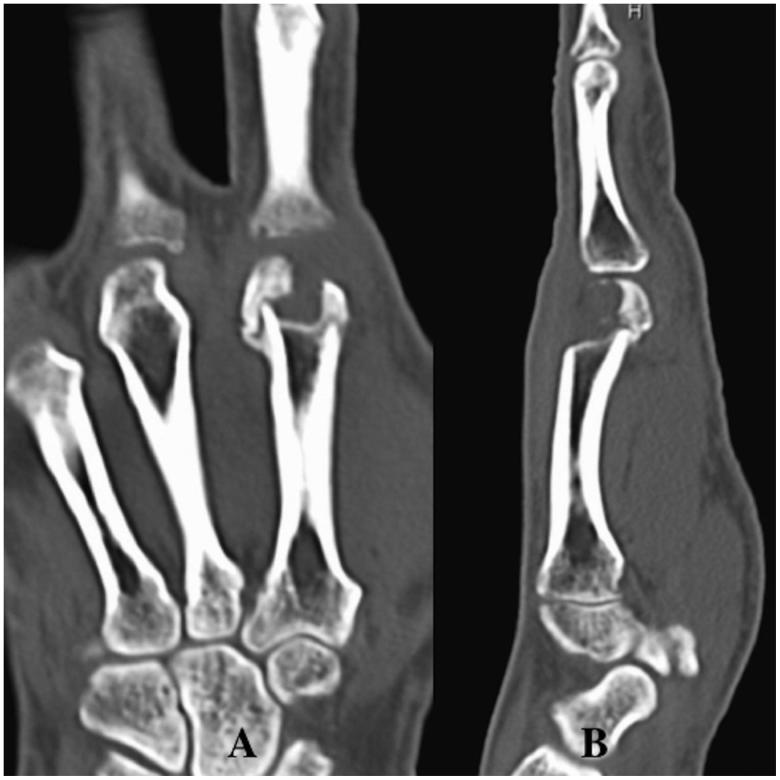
Case 1. (A and B) CT images showing the second metacarpal head defect.
